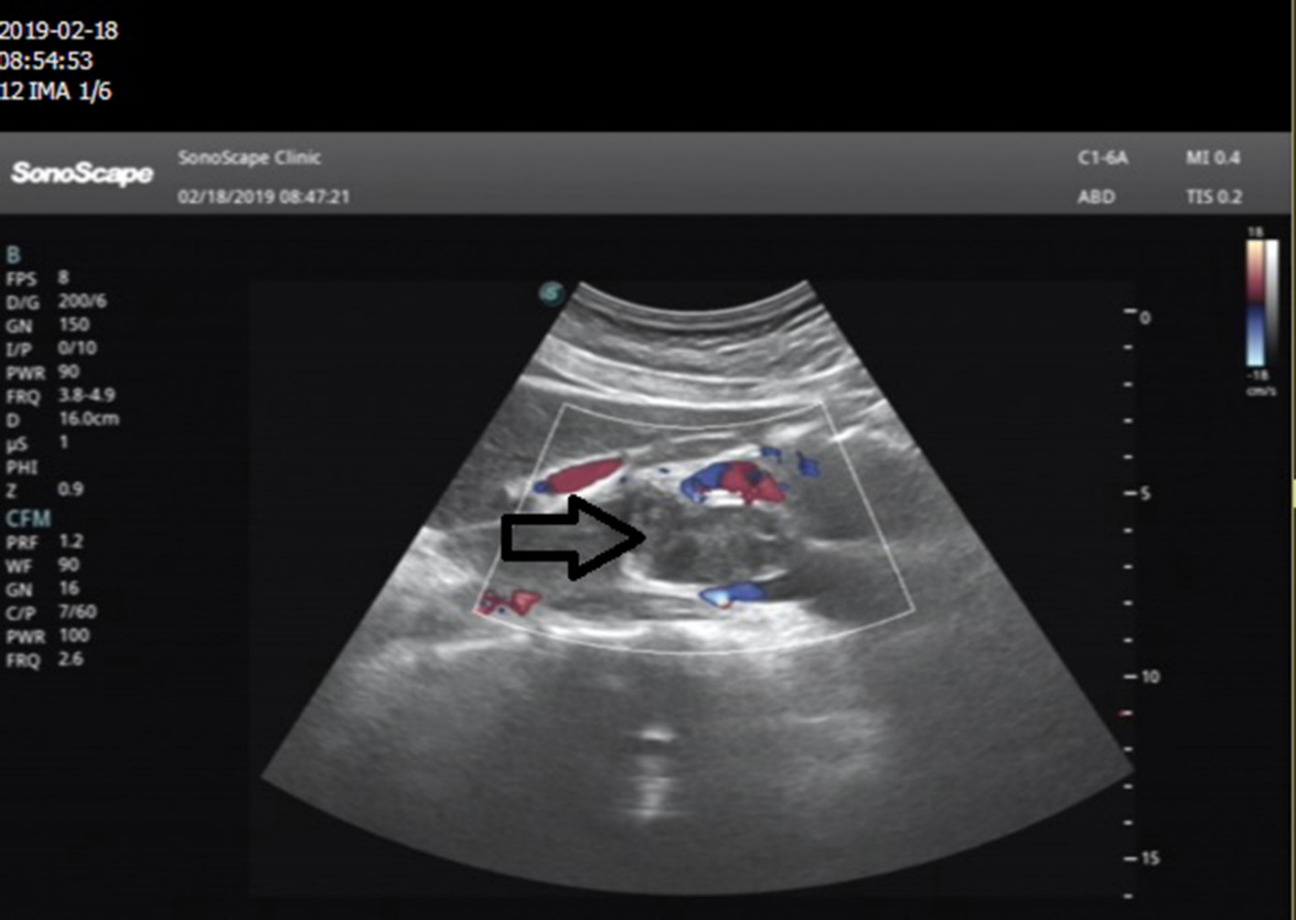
Abdominal ultrasonography images in the epigastric sagittal view: a hypoechoic, irregularly shaped solid mass was seen adjacent to the IVC.
